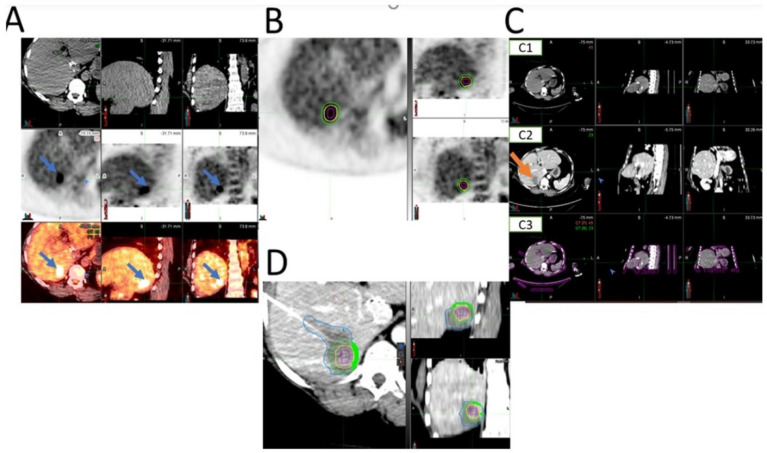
Steps in assessment of intraprocedural PET/CT guided ablation. (A) Accurate detection of tumor on pre-ablation imaging modalities is the first step (here, the tumor was detectable on PET and PET/CT—shown with blue arrows—but not on CT alone). (B) Segmentation of tumor (red circle) and margins (5−mm margin depicted with yellow circle and 10−mm depicted with green circle) on pre-ablation PET. (C) Registration of pre-ablation non-contrast CT (C1) and post-ablation portal venous CT (C2) (registration is shown in C3). The ablation zone is shown with an orange arrow on the portal venous phase of the post-ablation contrast-enhanced CT (C2). (D) The tumor (red circle), 5−mm (yellow circle), and 10−mm (green circle) margins transferred from pre-ablation PET to the registered post-ablation portal venous CT for assessment of the tumor and tumor margins that are not covered within ablation zone AZ (AZ is depicted with blue here; the margins that are not covered by the AZ are filled with green).
